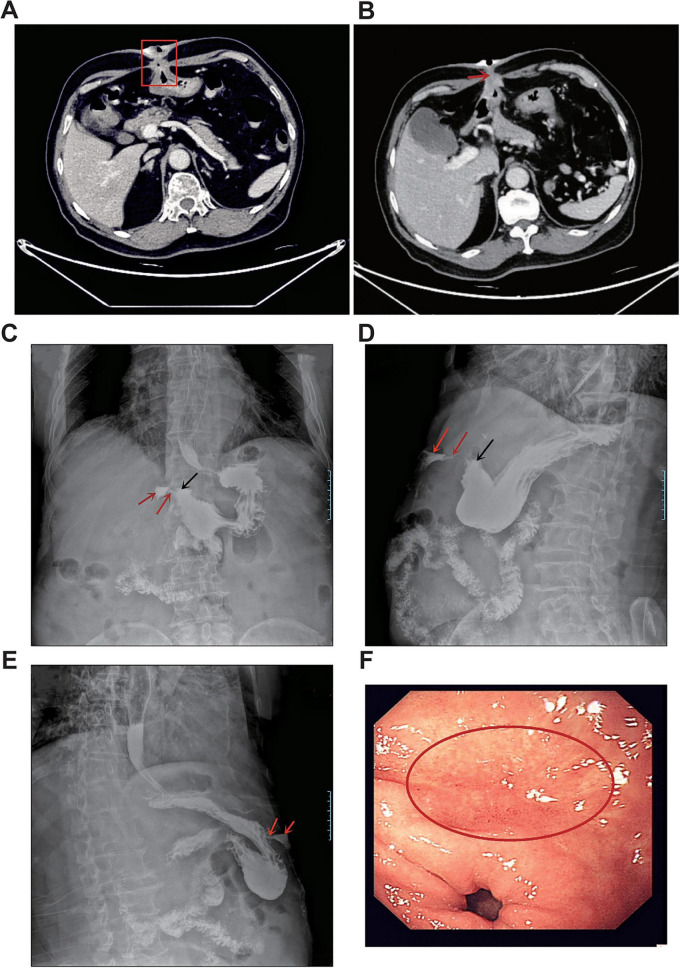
Preoperative imaging and endoscopy. (A,B) Contrast-enhanced CT scans. The red box (A) and red arrow (B) highlight the contiguous gastrocutaneous fistulous tract. (C–E) Upper GI contrast study in anteroposterior (C), left lateral (D), and right lateral (E) views, showing contrast extravasation from the gastric antrum (black arrows) through the fistula (red arrows). (F) Esophagogastroduodenoscopy (EGD) revealing the internal orifice; the red circle indicates an irregular ulcer (1.2 × 0.4 cm) on the antral lesser curvature.
